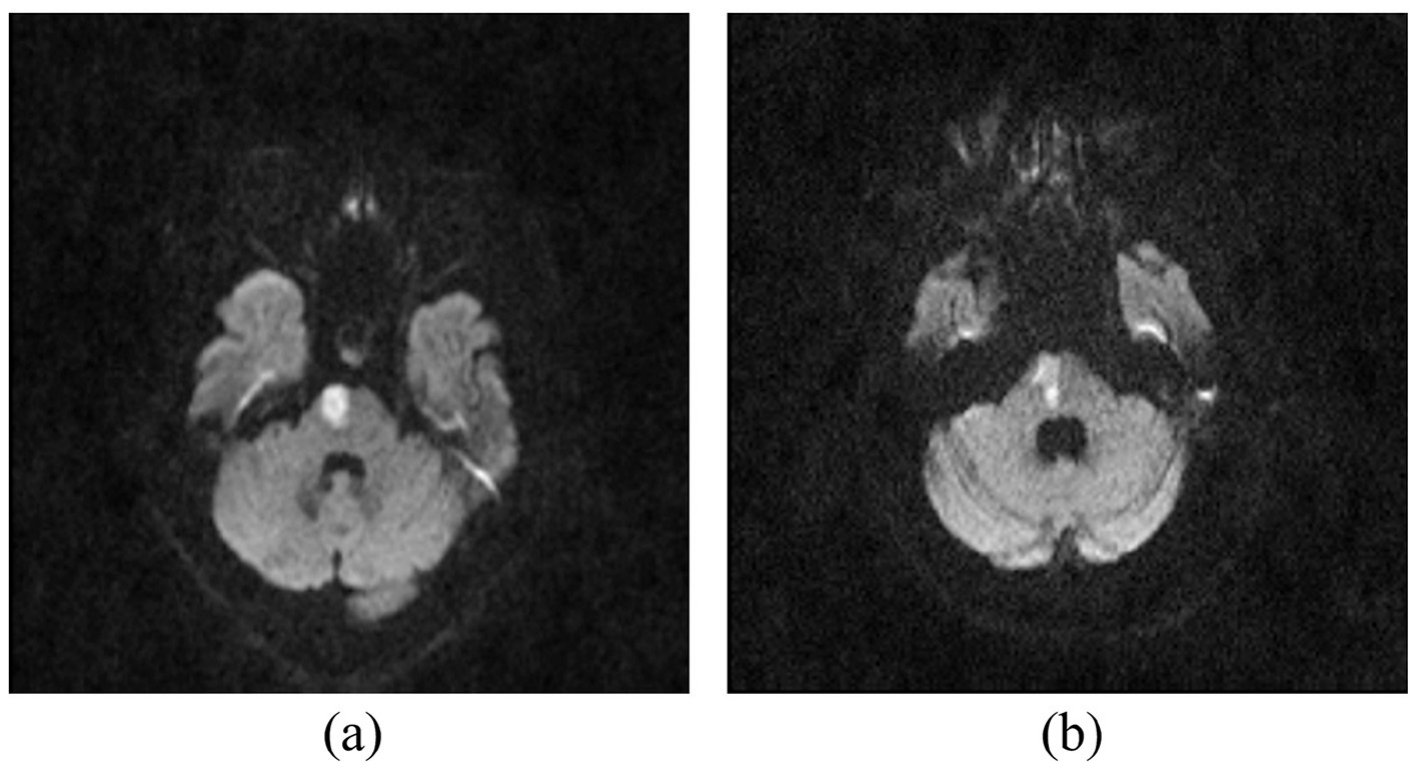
The MRI slices from the dataset, where (a) shows a sample slice from an ND case, and (b) presents a slice from a non-ND sample.
